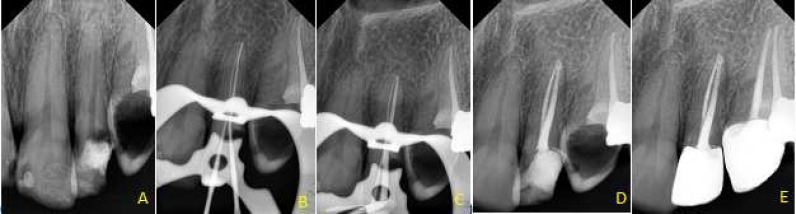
A)* Preoperative periapical radiography of the maxillary left lateral incisor; *B)* radiography taken after detecting the root canals; *C)* Radiography taken for evaluation of master cone; *D)* Periapical radiography taken after completion of root canal filling; *E)* Follow-up radiography
